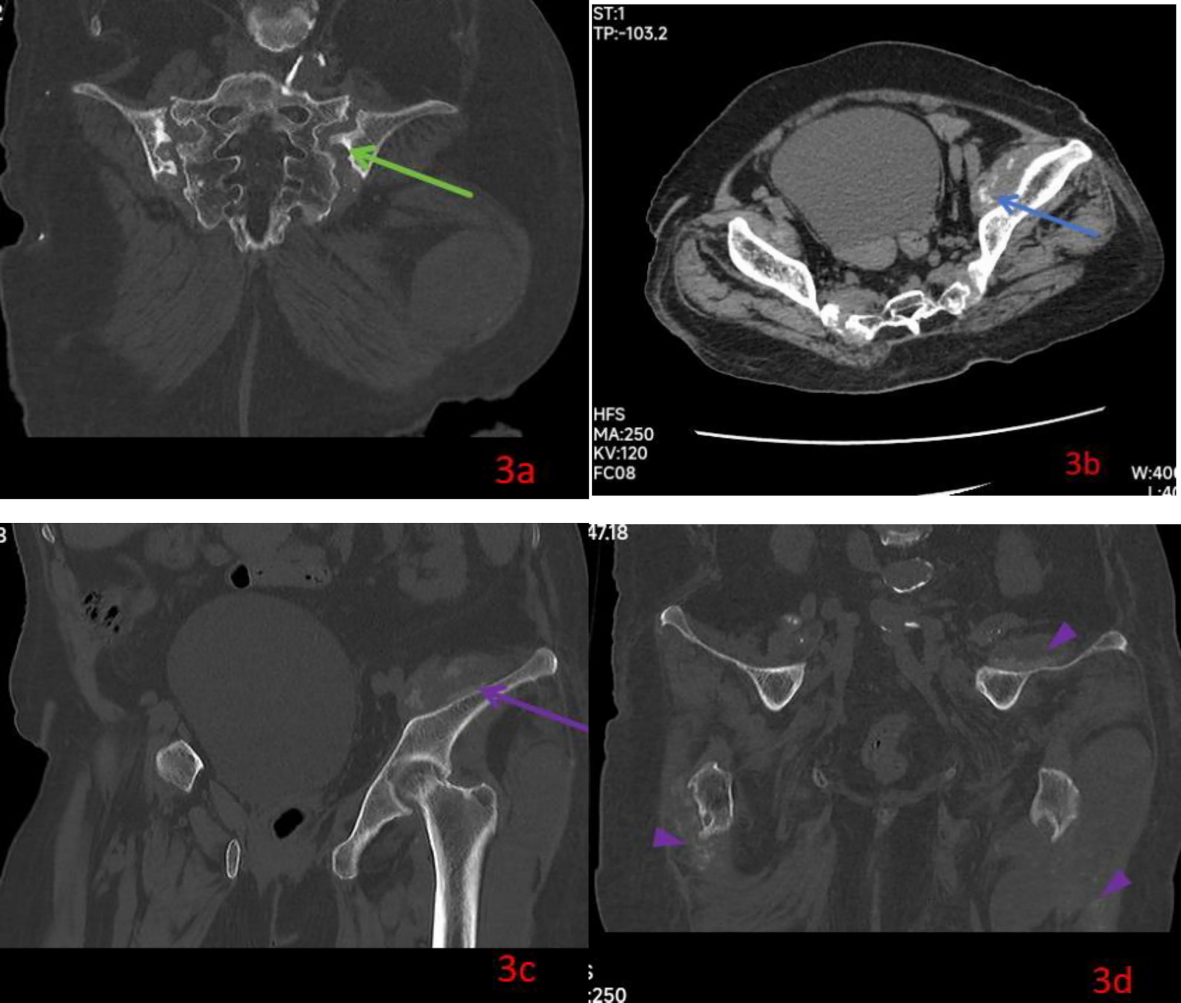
Coronal view of pelvis CT (a) after admission showed erosion of the sacral and iliac borders with widening of the left sacroiliac joint and condensation of the adjacent bone green arrow; Horizontal view (b) of pelvis CT showed a multilocular abscess within the left psoas muscle (blue arrow); Coronal view of pelvis CT (c,d) showed a multilocular abscess within the left psoas muscle (purple arrow) and juxta-articular soft tissue masses containing sandy calcification around pelvis and both hip (purple triangle).
